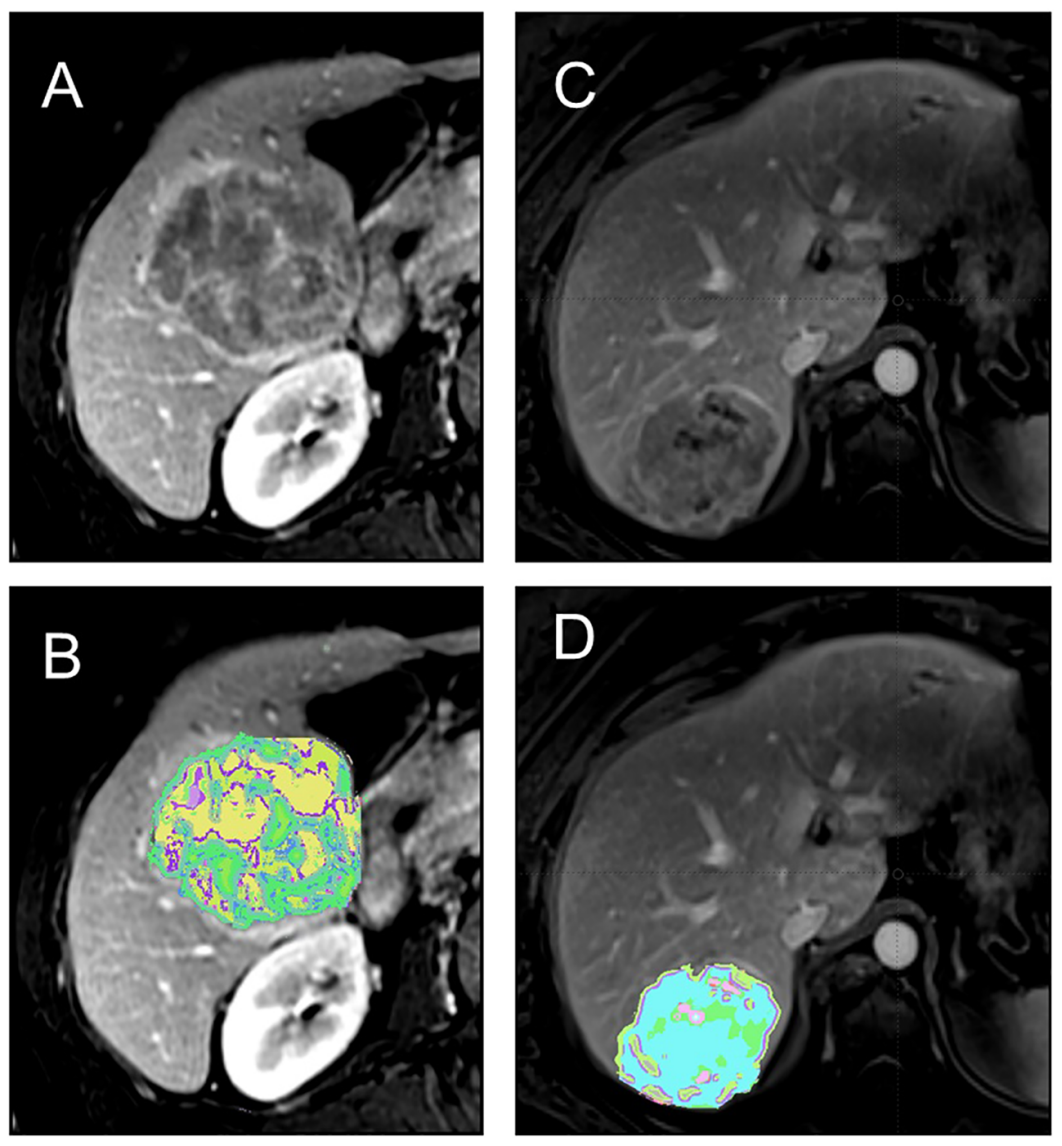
(A, B) 64 y/o M with history of cirrhosis and HCC.Contrast-enhanced T1-weighted MRI shows a heterogeneous tumor (A) with associated texture heterogeneity map demonstrating tumor habitats (B). The patient had poor outcome with several recurrent lesions after surgical resection suggestive of poor tumor biology. (C, D) 54 y/o F with history of cirrhosis and HCC. Contrast-enhanced T1-weighted MRI shows a less heterogeneous tumor (C) with associated texture heterogeneity map showing the tumor habitats (D). The patient good outome after resection with no recurrence.
